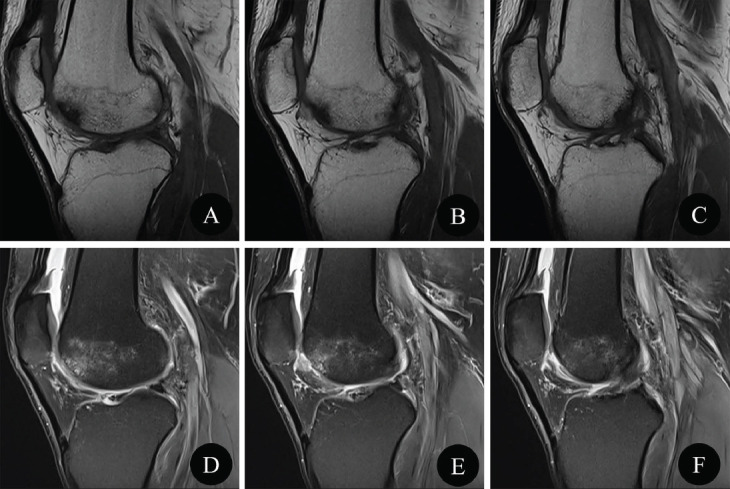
Second presentation of the right knee MRI examination. Sagittal T1WI (A-C) and PDWI-fs (D-F) showed local cartilage fracture and defect of the lateral femoral condyle, subchondral bone marrow edema, and joint effusion, while no cartilage loose body was clearly detected.
